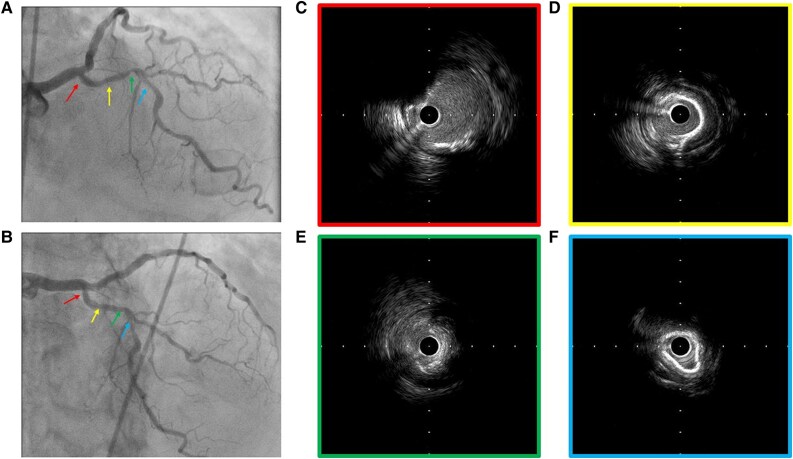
(*A* and *B*) Angiography images showing severe disease in the proximal to mid-left anterior descending artery, with marked tortuosity and heavy calcification. Arrows indicate anatomical locations within the proximal left anterior descending artery corresponding to intravascular ultrasound images. (*C*) Eccentric wire bias adjacent to calcified plaque (arrow). (*D*) Eccentric calcified lesion (arrow). (*E*) Soft plaque within a tight lesion (arrow). (*F*) Concentric calcified lesion (arrow).
